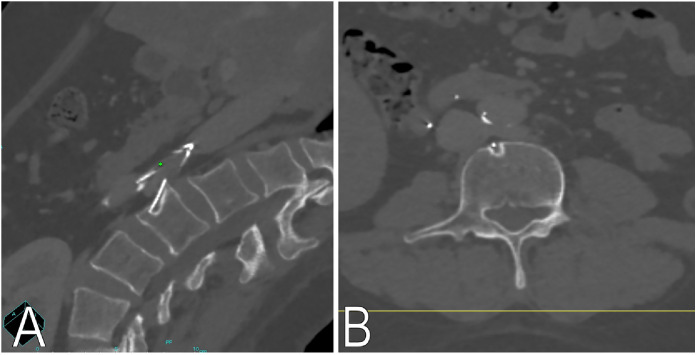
Multi-projection reconstruction images oriented (A) and axial non-contrast CT images (B) showing a filter strut penetrating the anterior L3 vertebral body with chronic cortical scalloping.
